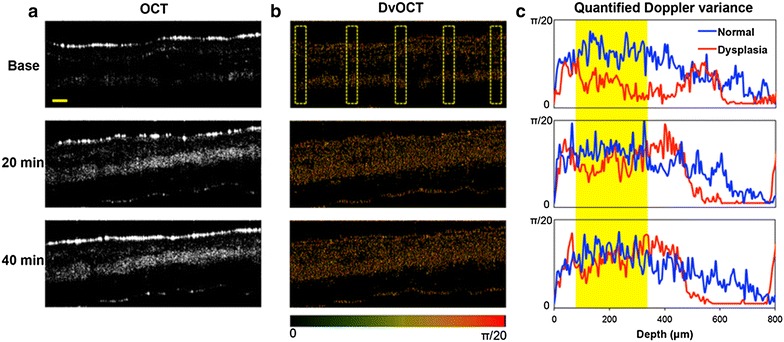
OCT and DvOCT imaging of dysplastic hamster check pouch with non-acid-disassembling Au NCs. Images of DMBA-applied, early-stage dysplastic hamster cheek pouches, obtained by SD-OCT (a) and DvOCT (b) after administering non-acid-disassembling Au NCs. Scale bar: 100 µm. The images were taken before (base) and 20 and 40 min after administering non-acid-disassembling Au NCs. The color-coded Doppler variance in DvOCT images of early-stage dysplasia (5 dotted regions) was quantified and compared with that of normal tissue (c) (yellow area: epithelial layers)
